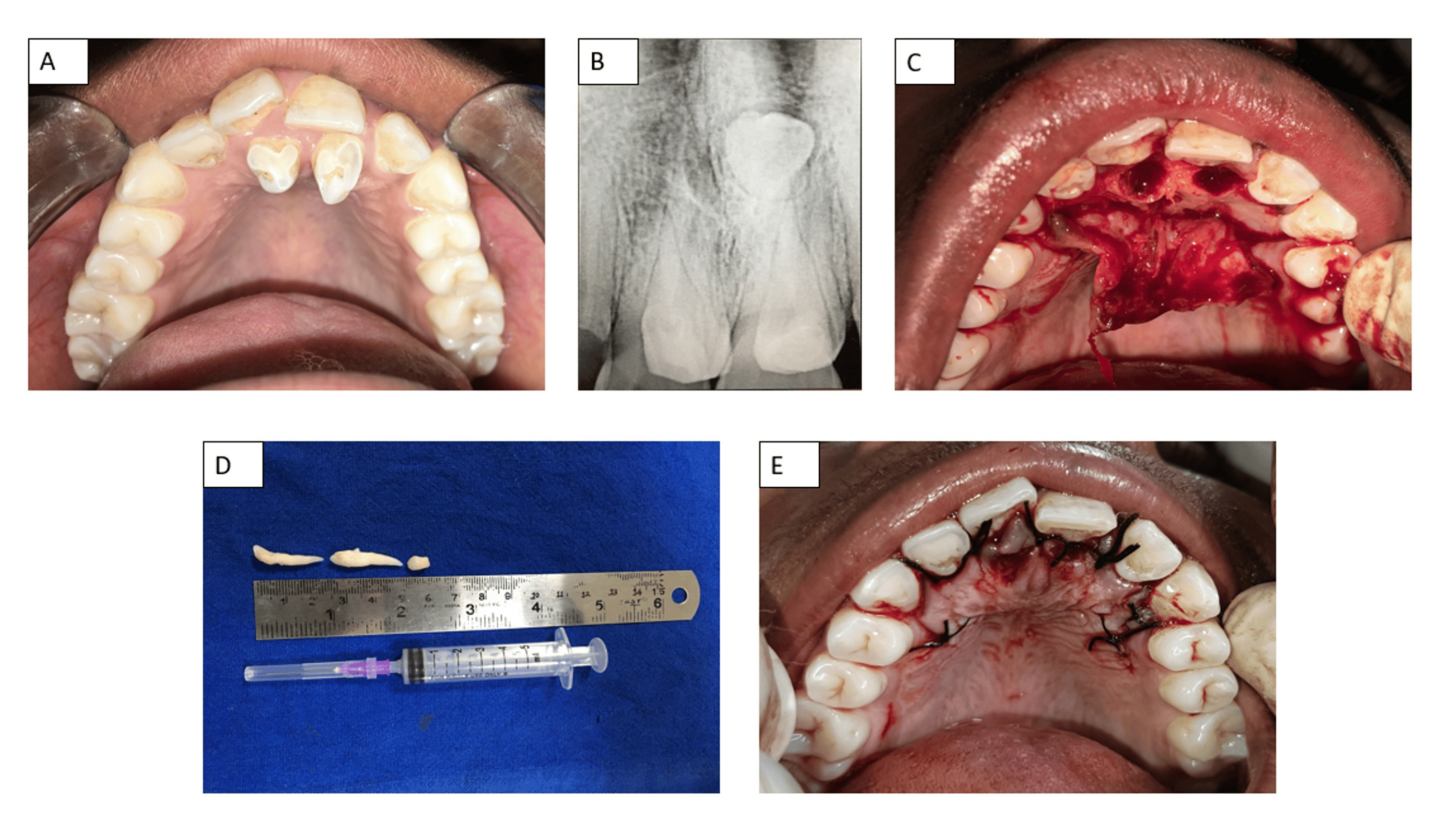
(A) Preoperative view of maxillary anterior supernumerary teeth (tuberculate type); (B) Intra-oral periapical radiograph showing three supernumeraries; (C) Palatal full-thickness mucoperiosteal flap raised; (D) Extracted three supernumerary teeth; (E) Postoperative suturing with 3-0 silk sutures
